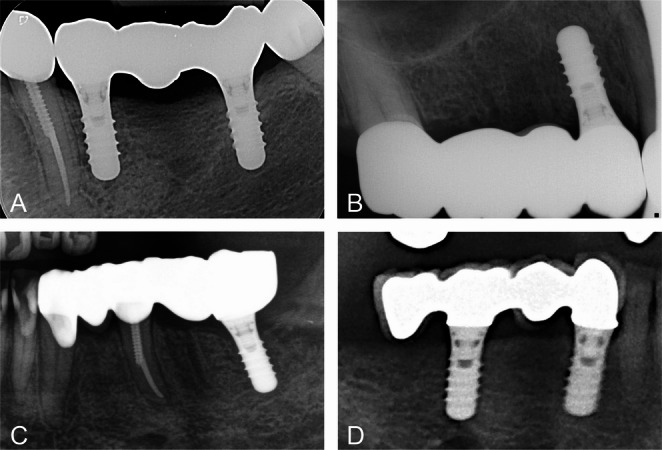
Radiographs of exemplary fixed dental prostheses (FDP). A, Implant supported FDP with 3 units and a unit/abutment relation of 1.5. B, Combined toot-implant supported FDP with 4 units and a unit/abutment relation of 2. C, Combined toot-implant supported FDP with 5 units and a unit/abutment relation of 1.67. D, Implant supported cantilever FDP with 4 units and a unit/abutment relation of 2
